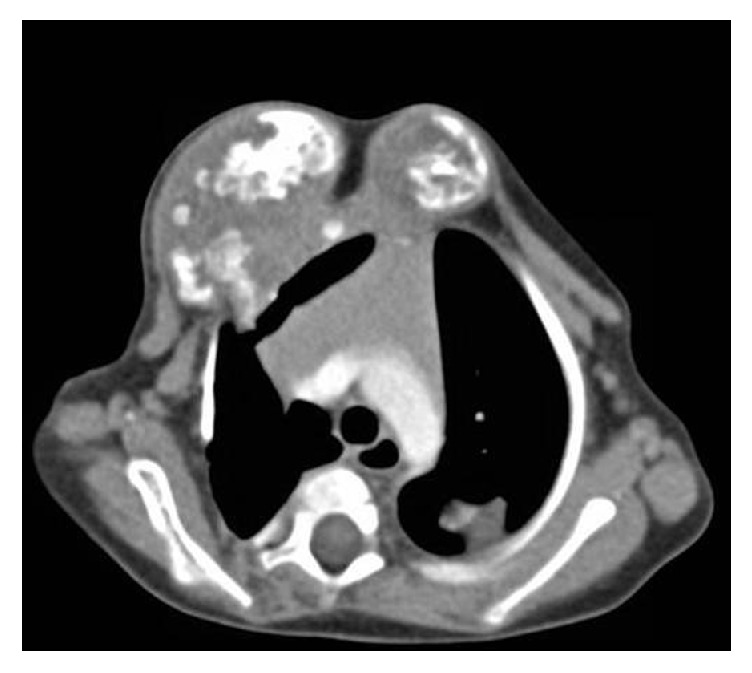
This contrast chest CT scan shows multiple bilateral masses involving mainly the upper ribs, extending into the thorax and causing mediastinal shift to the left. The largest mass measuring 5,7 cm × 4,1 cm is located on the right side. The masses consist of large multiloculated soft tissue lesions with calcifications. The appearance is mostly suggestive of a chest wall bone tumor with likely soft tissue metastasis.
